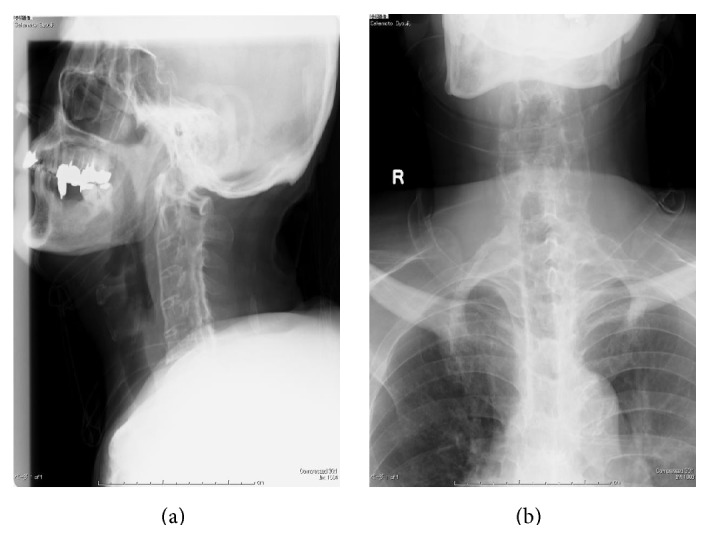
Plain radiographs of the cervical spine were unremarkable in detecting a cervical spine fracture in a patient with AS although the typical radiographic features of AS were apparent in entire spine ((a) AP and (b) lateral).
